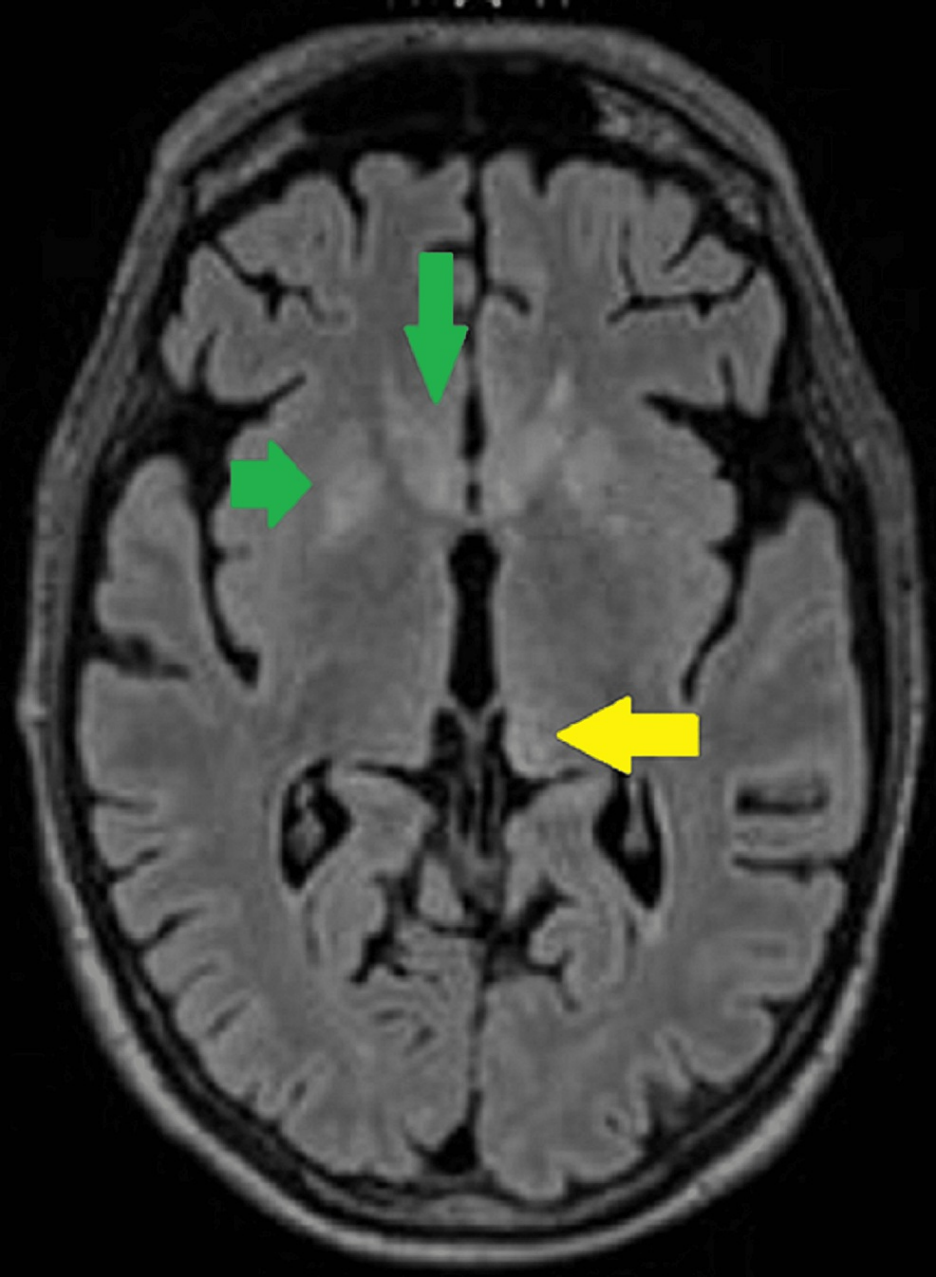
MRI Brain Fluid-attenuated inversion recovery (FLAIR) axial image showing hyperintense signals in the head of bilateral caudate nuclei, putamen (green arrows), and dorsomedially in the left thalamus (yellow arrow).
